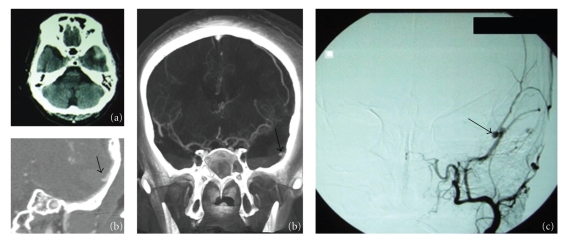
Patient with traumatic intracerebral hematoma: in (a) skull computed tomography with temporal hematoma, in (b) angiotomography with hematoma and pseudoaneurysm, and in (c) digital angiography confirms a pseudoaneurysm in middle meningeal artery.
